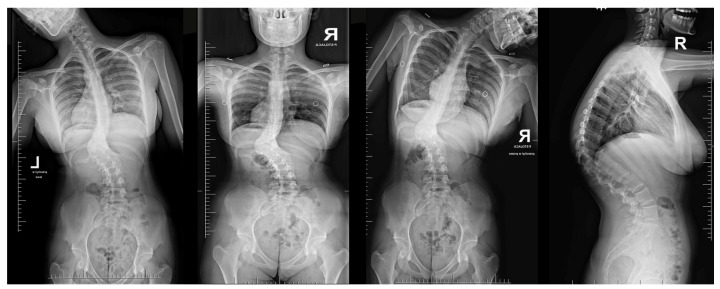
Standard standing AP and lateral X-rays and side-bending films of the 16-year-old female before surgical treatment. These X-rays showed severe and stiff congenital kyphosis.
